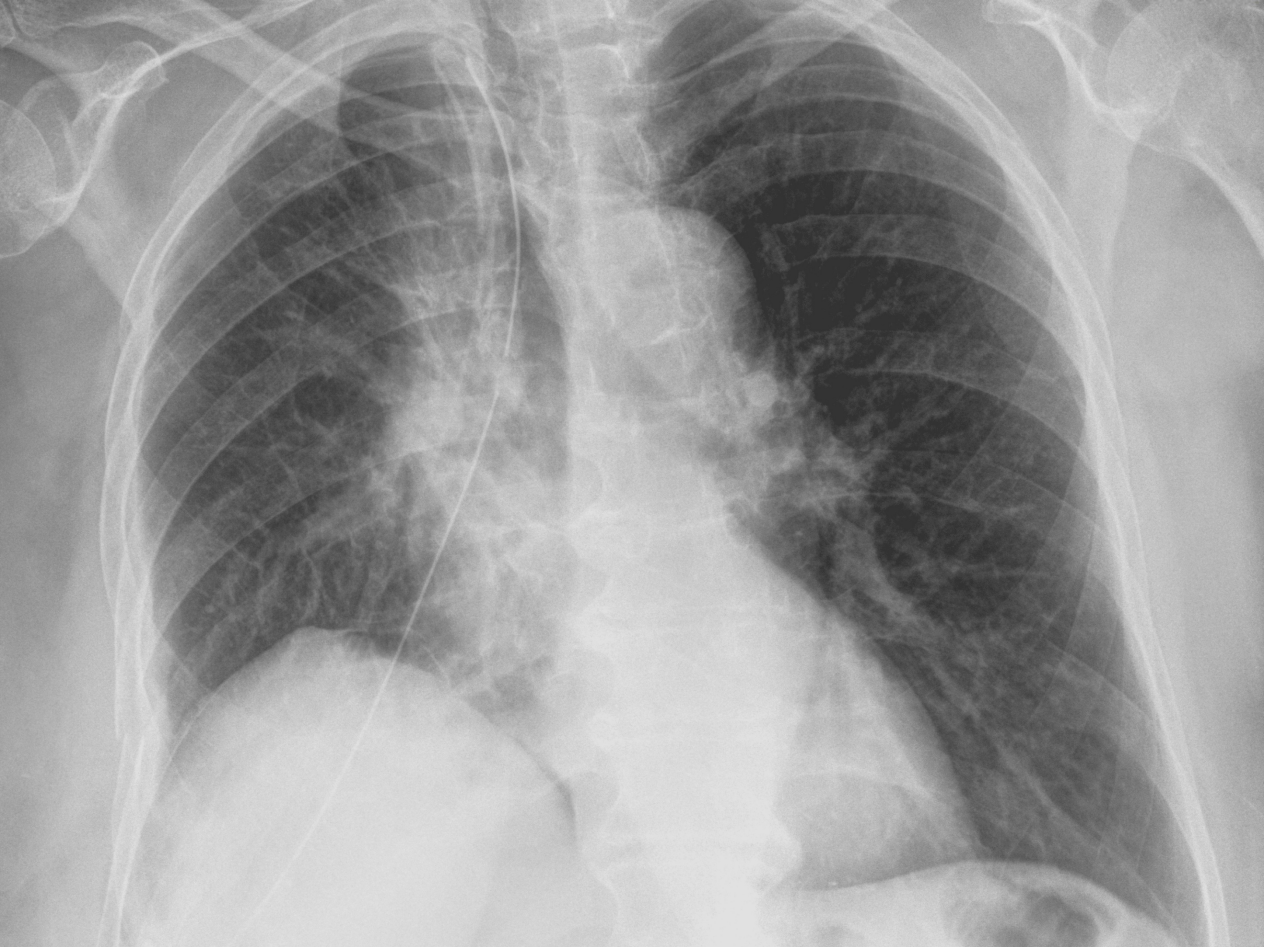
Chest x-ray on the 6th postoperative day just before removal of the thoracic catheter.
